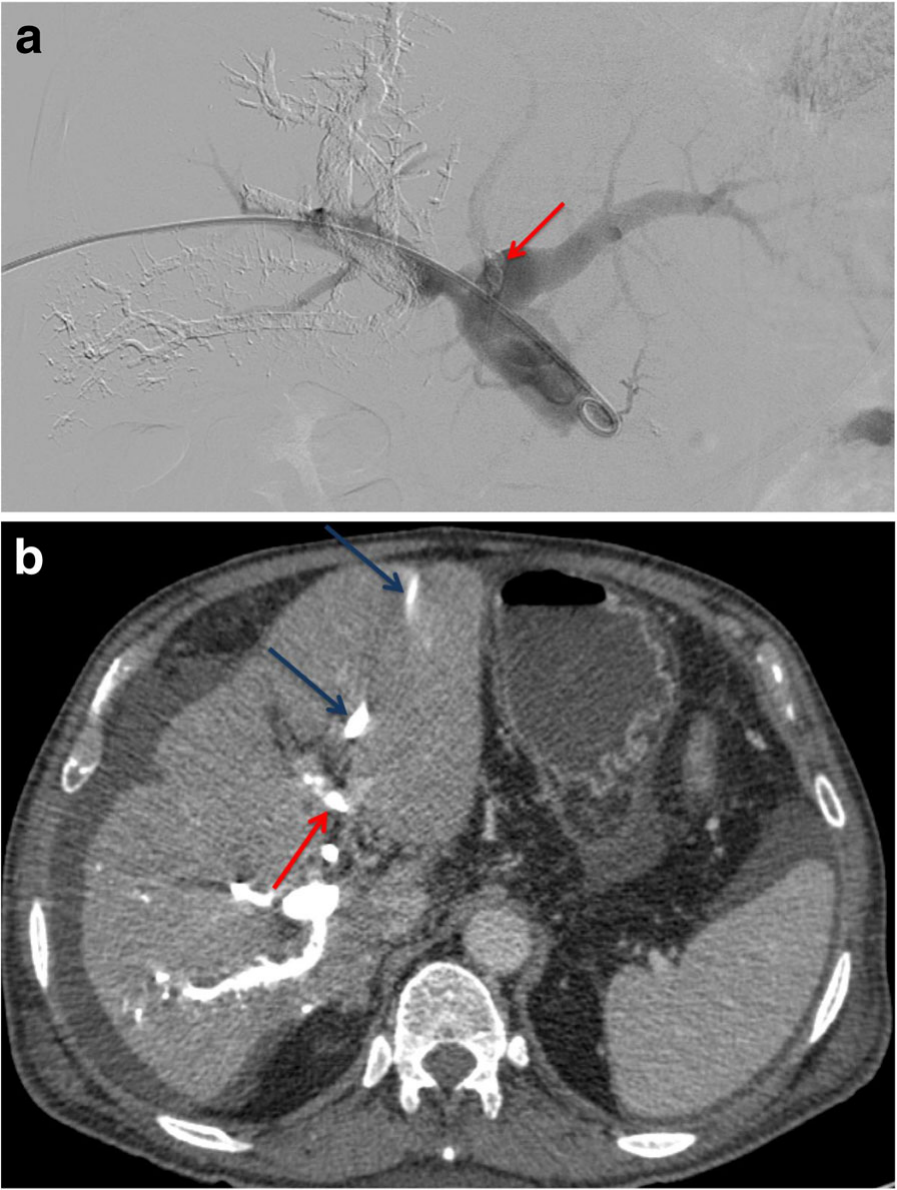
a Portography showing the dislodged NBCA. Direct portography during PVE showing the dislodged NBCA fragment in the left portal vein (red arrow). b CT showing dislodged NBCA. Contrasted-enhanced CT 4 weeks after PVE showing the NBCA fragment in the left portal vein (red arrow). This patient presented a 20% FLR hypertrophy but deceased due to fulminant cholangitis before surgery. (dark-blue arrow - Biliary drain trajectory in the liver)
